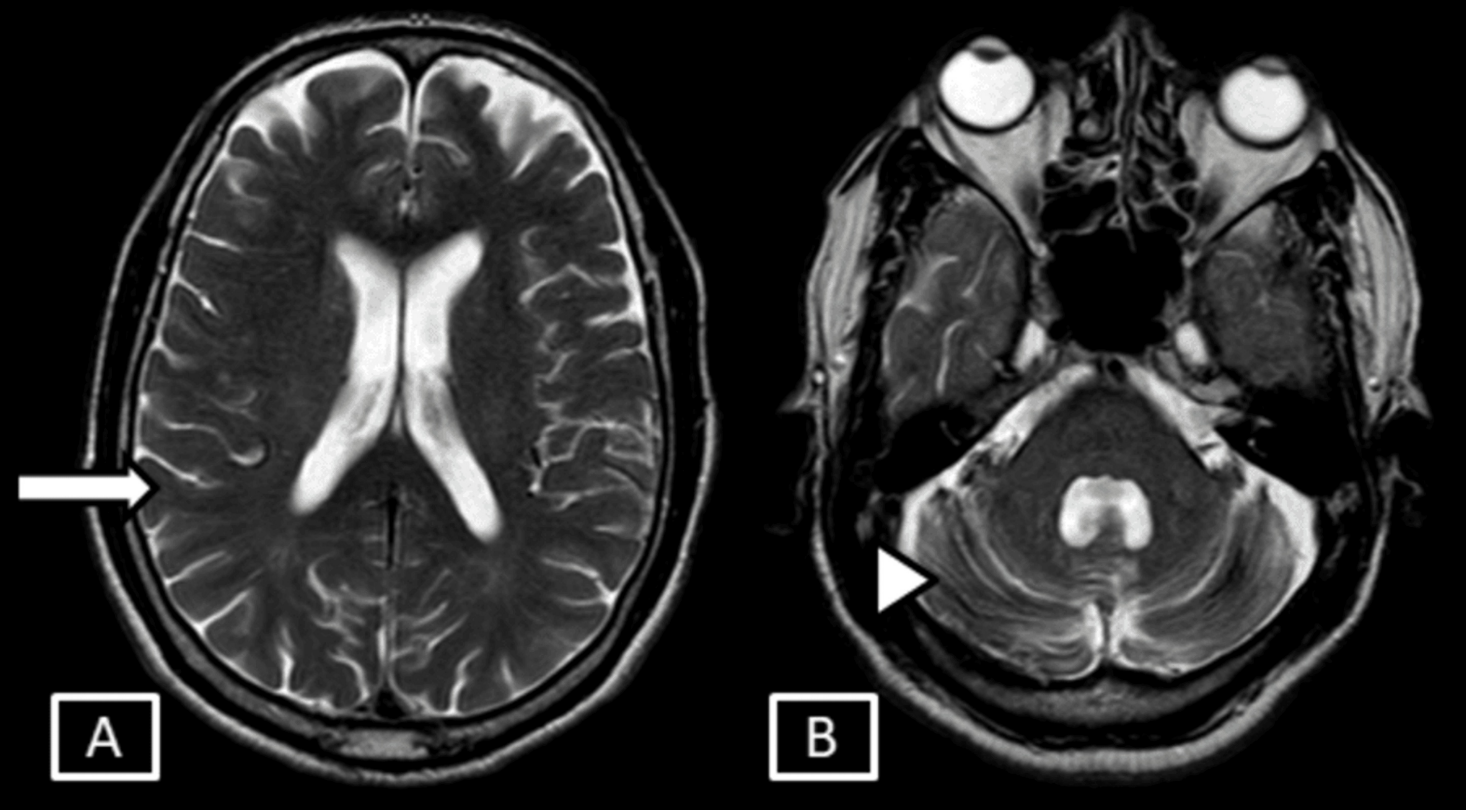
Case 2 MRI brain T2 axial images. Images show mild age-related degenerative changes in the form of prominent sulcal, extra-axial CSF spaces (A) represented by the white arrow and along the cerebellar foliae (B) represented by the white arrowhead. CSF: cerebrospinal fluid
